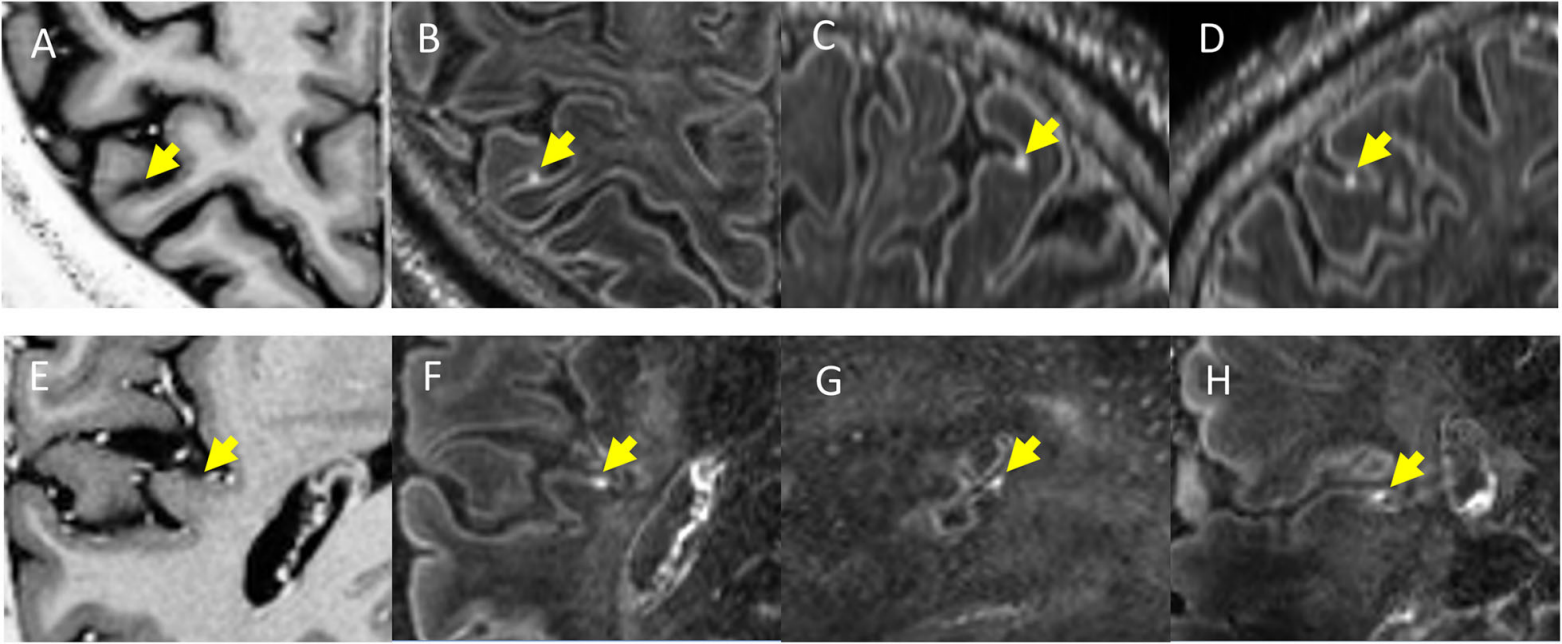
Examples of “nodular” type enhancing foci in multiple sclerosis. In the horizontal rows are displayed two different patients with multiple sclerosis. T1-weighted magnetisation-prepared two rapid gradient echo images (A, E), along with axial (D, H), sagittal (C, G), and coronal (B, F) slices from contrast-enhanced magnetisation-prepared fluid-attenuated inversion recovery images. The yellow arrows indicate the location of a nodular focus of leptomeningeal contrast enhancement in all three planes and its expected location on magnetisation-prepared two rapid gradient echo images. Published with permission from *J Neurol* [53]
